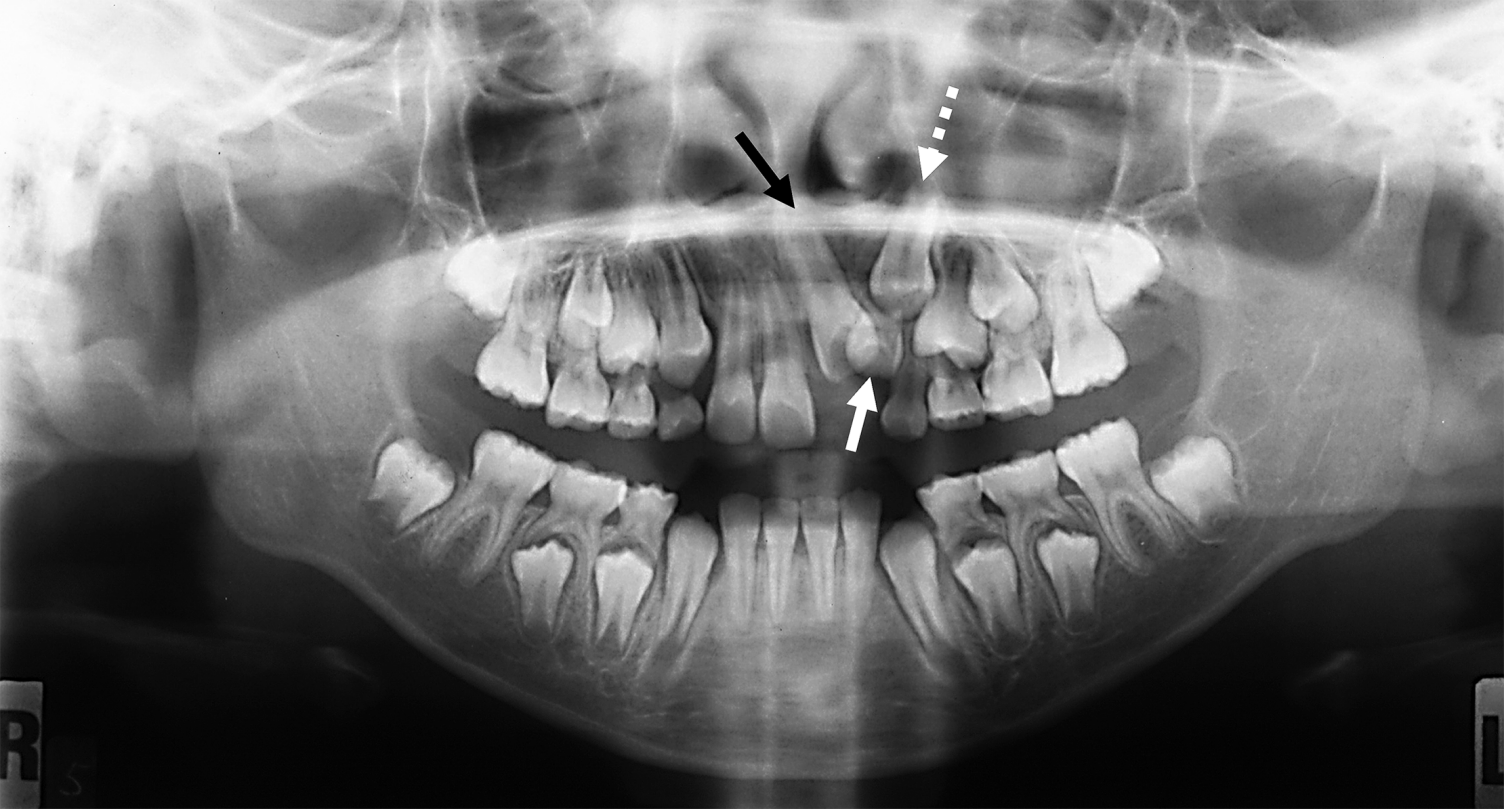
Panoramic radiograph of an 8-year-old boy showing the unerupted #10 with delayed root development (white arrow), which also acted as an obstruction to the eruption of #9 (black arrow) and #11 (dotted arrow).
